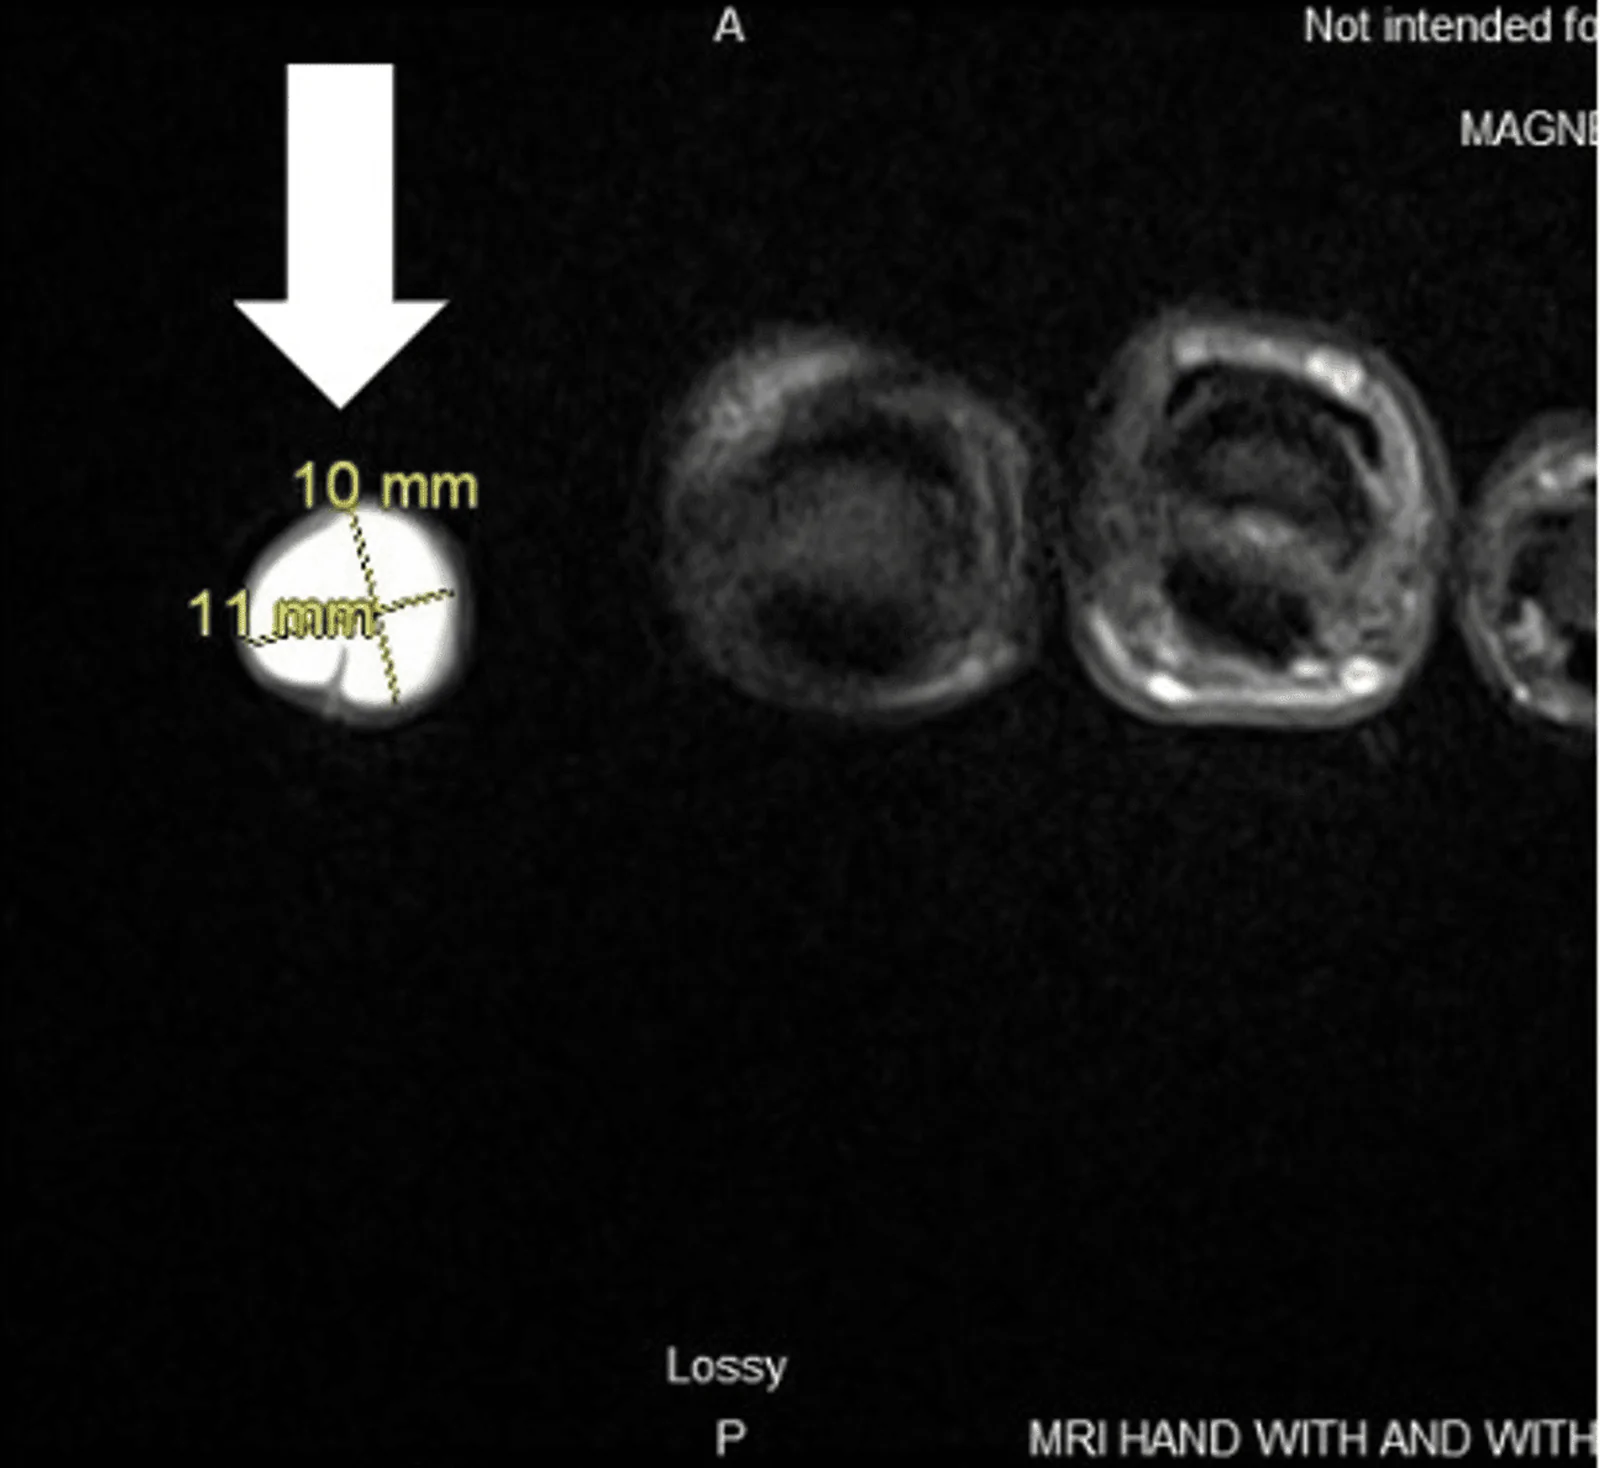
Coronal PD FS magnetic resonance image showing homogeneous high signal intensity of the lesion, leading to a preliminary radiologic impression of epidermal inclusion cyst. PD FS: proton-density fat-saturated
